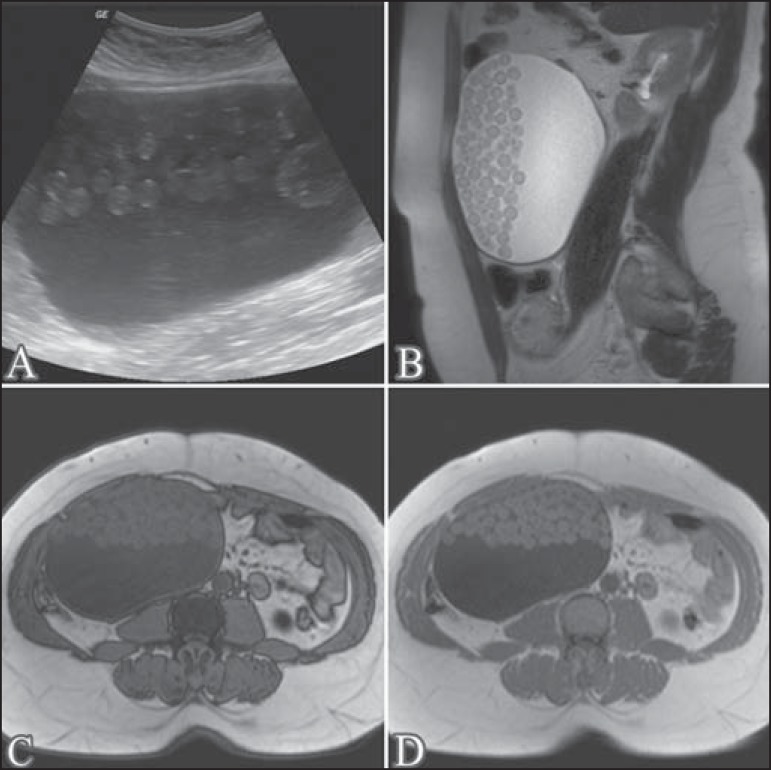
Ultrasound (A); sagittal T2-weighted MRI sequence
